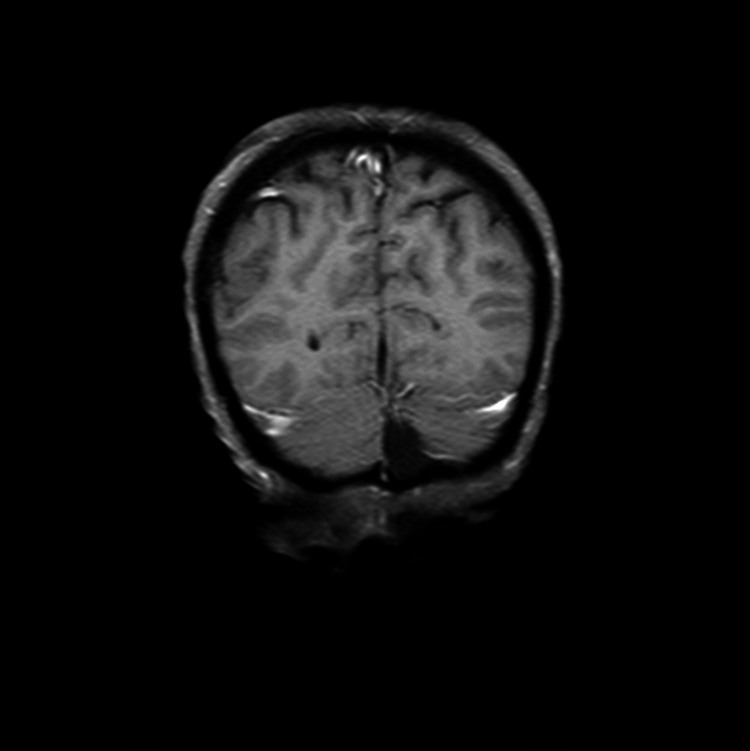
T1-weighted coronal brain magnetic resonance image with contrast on April 25, 2019
